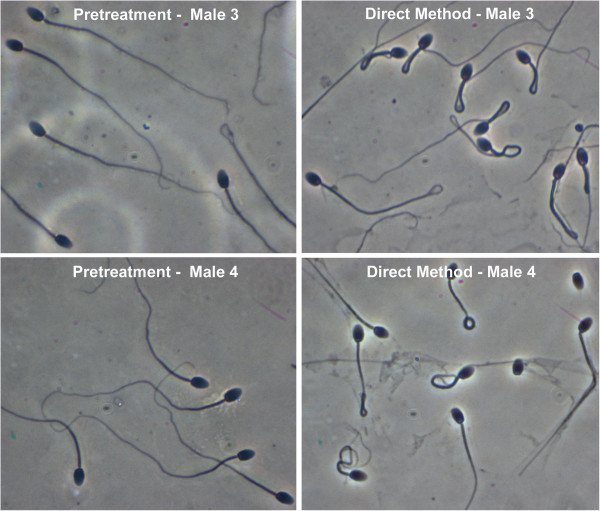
Morphology of sperm from males before or after the direct method of ultrasound treatment.
